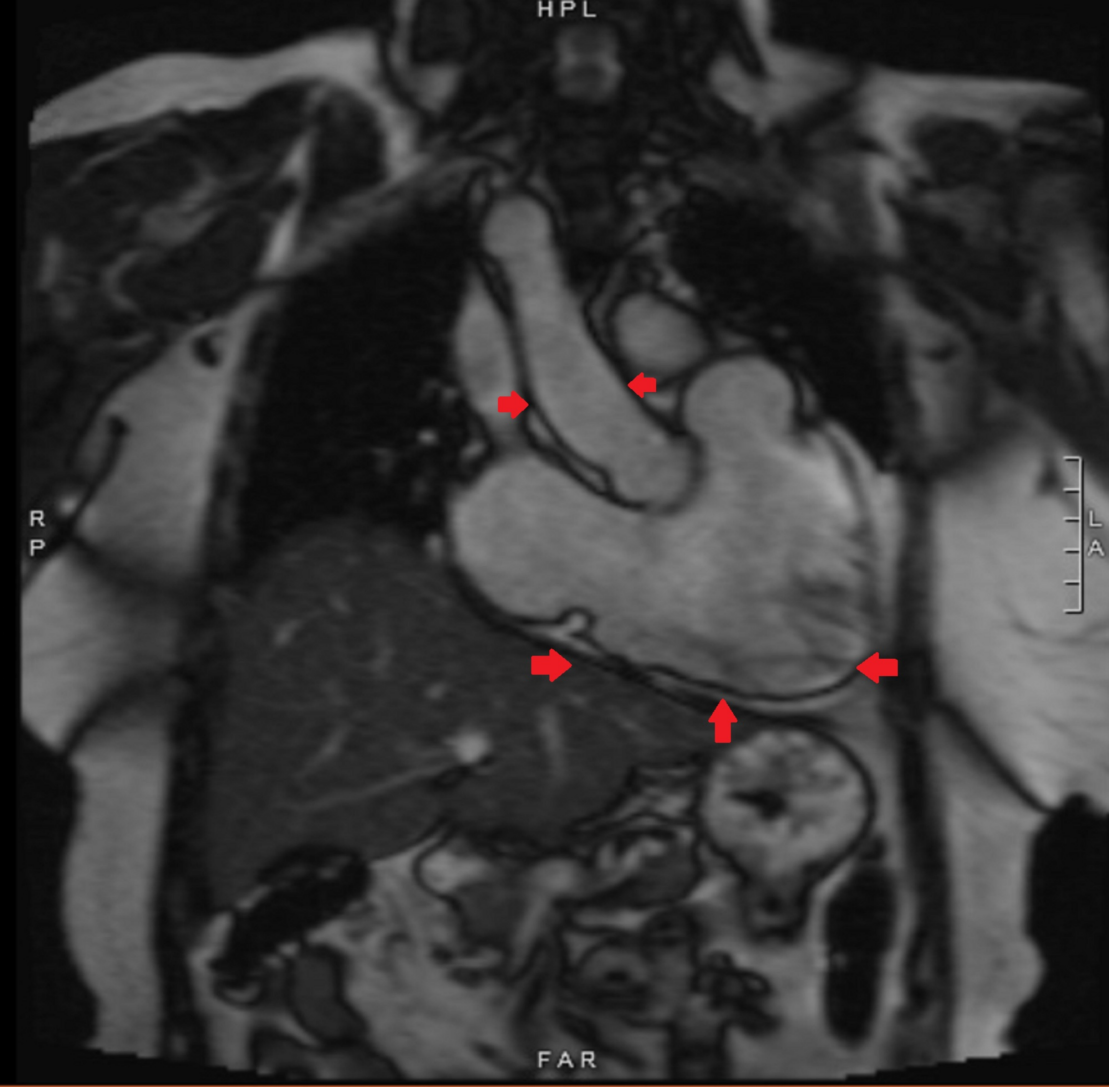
Preoperative cardiac magnetic resonance imaging showing dilated right ventricle and right ventricular outflow tract
